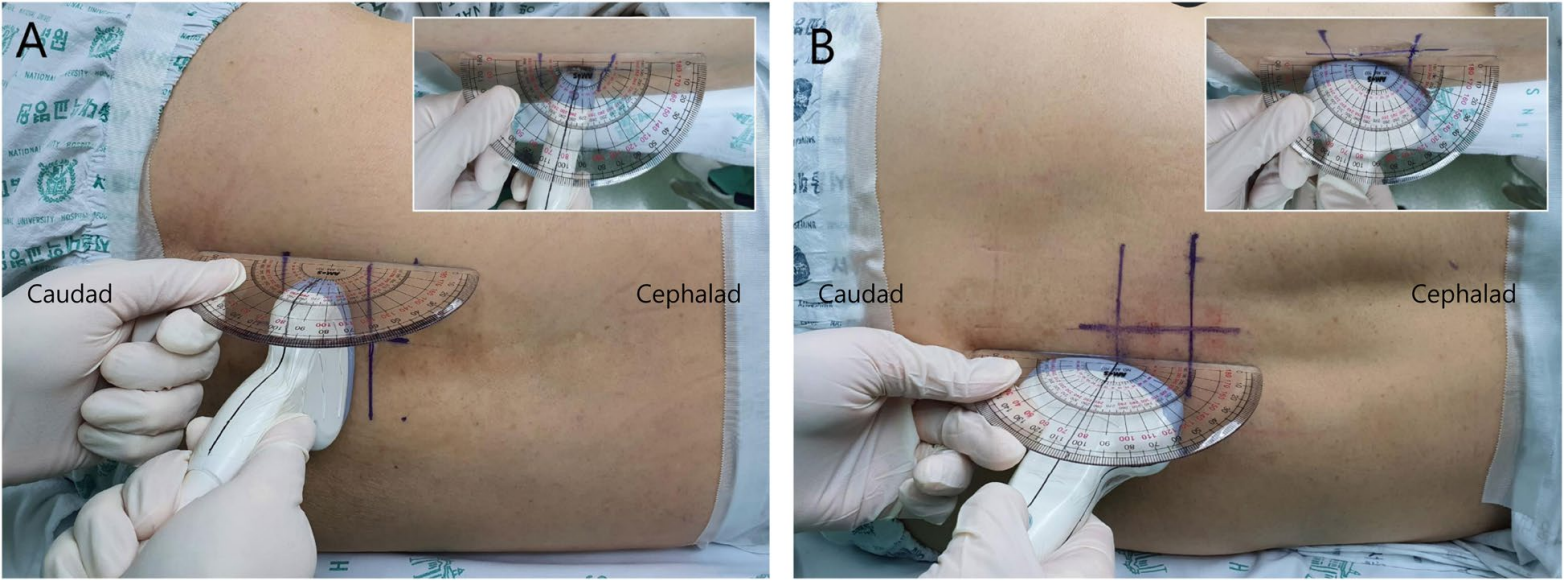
The method of angle measurement at transverse midline view and parasagittal oblique view. (A) The ultrasound probe was placed transverse to the patients' back and was tilted toward cephalad or caudad to find the longest observed the ligamentum flavum-dura mater complex (LFD) height. The probe application angle with the longest interlaminar height of LFD was measured using a protractor. The measured angle was ( +) 14° in this image. (B) The ultrasound probe was placed in the parasagittal plane with a medial tilt towards the midline. The probe is tilted toward cephalad to caudad, and the probe application angle with the longest interlaminar height of LFD was measured using a protractor. The measured angle was ( +) 15° in this image
